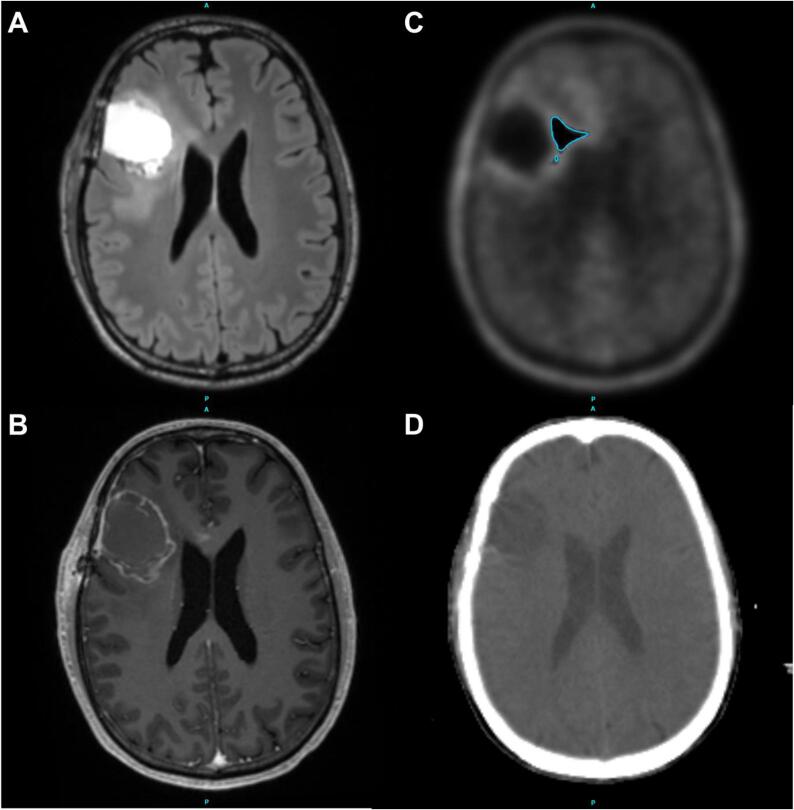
Representative screenshots of the sample patient's images sent to the study sites. A: MRI FLAIR, B: MRI T1, C: FET PET (BTV with TBR of 1.8), D: Planning CT. *(BTV = biological tumor volume; MRI = magnetic resonance imaging; FET = O-(2-[^18^F]fluoroethyl)-L-tyrosine; PET = positron emission tomography; TBR = target-to-background ratio; A = anterior; P = posterior).*
